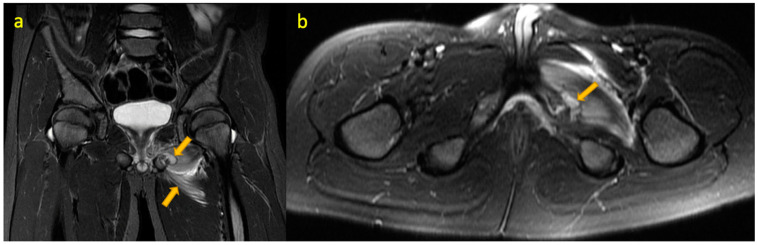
(a,b) Coronal STIR and axial T2W FS MR images of an 8-year-old boy with osteomyelitis of the left-sided ischiopubic synchondrosis. Note associated soft tissue abscess and muscle oedema.
